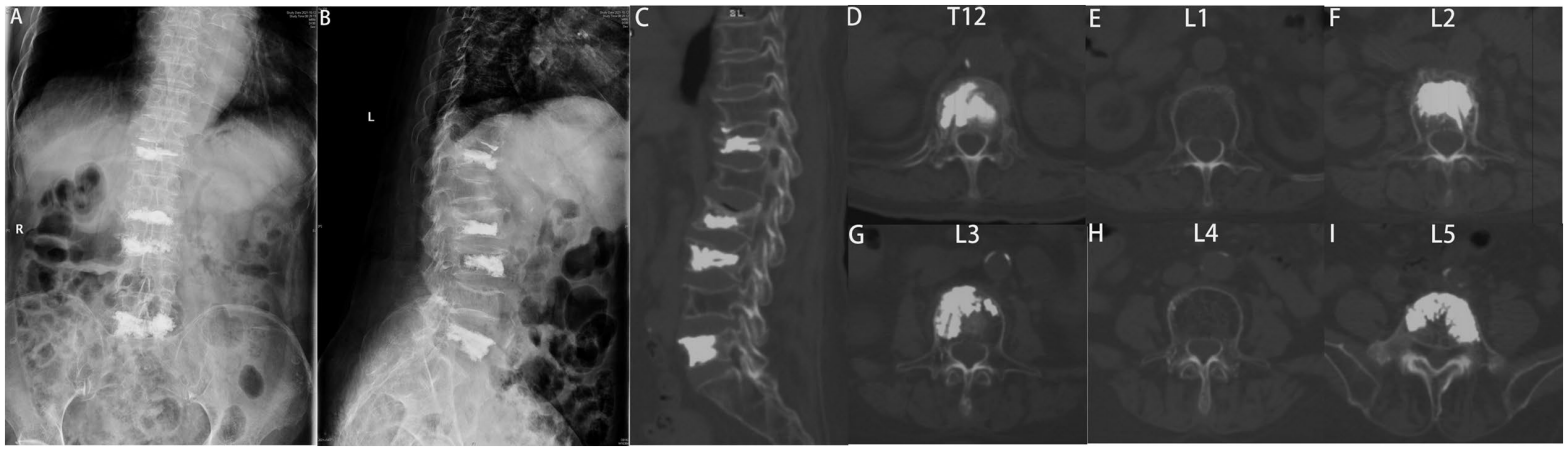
Postoperative X-rays of the spine. (A) Anterior–posterior and (B) lateral radiographs demonstrating successful bone cement filling without any leakage. The final follow-up CT scan of the spine (C−I) showing no evidence of new fractures.
